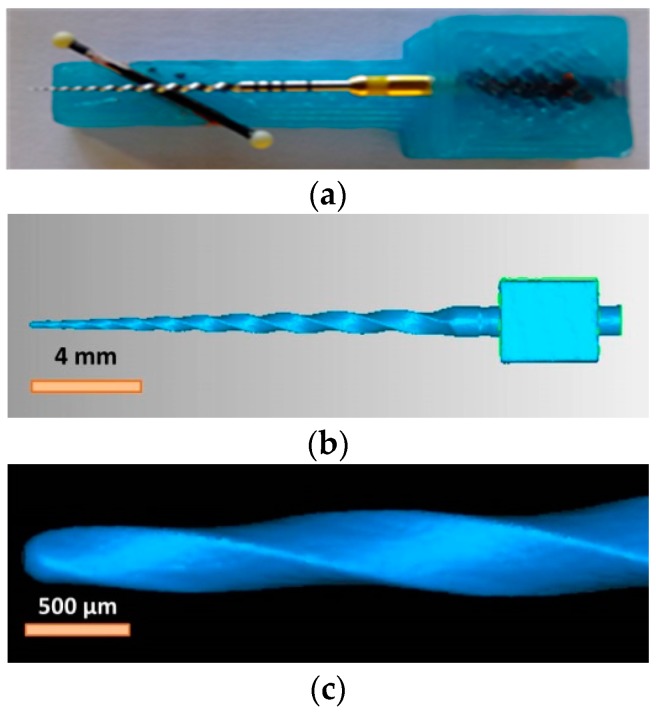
(a) Dental file and miniaturized ball bar during the measurement on the CT scanner; (b) 3D volume reconstructed from the CT scan of the complete dental file; (c) 3D volume reconstructed from the CT scan of the dental file tip and of the helix geometry.
